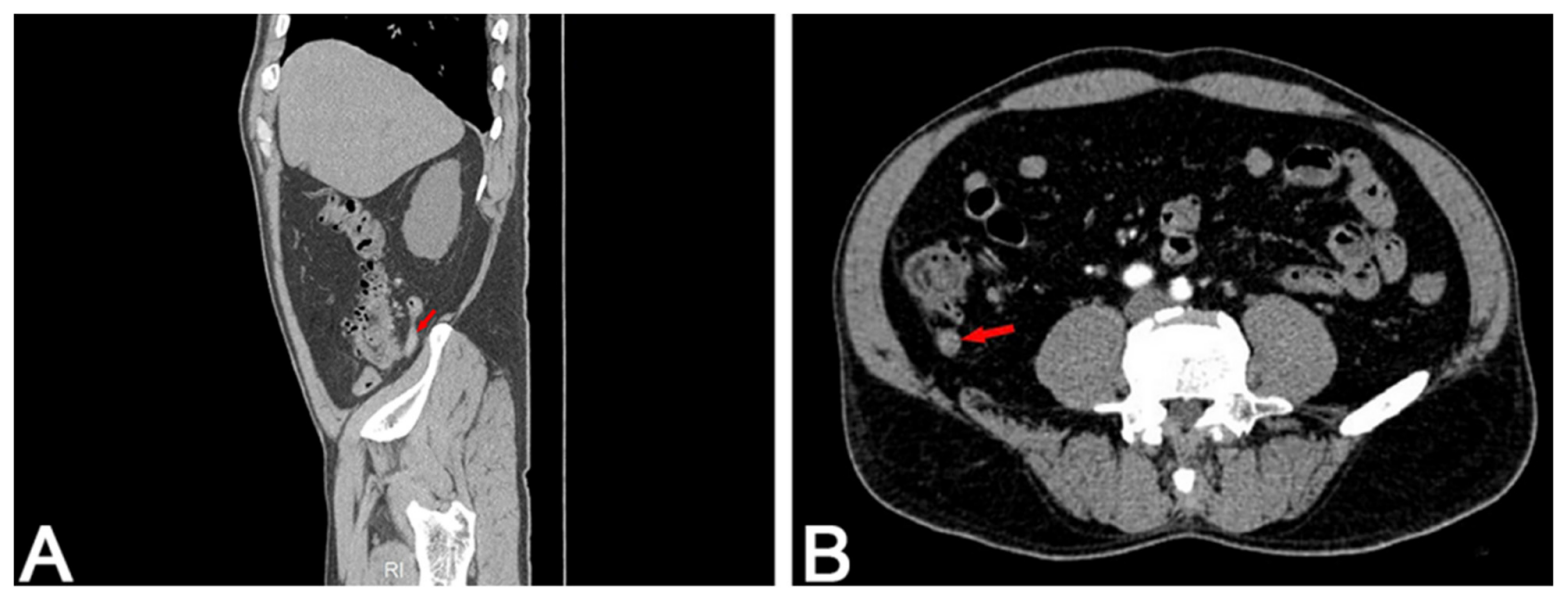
Contrast-enhanced abdominal CT scan. (A) Sagittal reconstruction shows an enlarged appendix with a maximal diameter of approximately 10 mm. (B) Axial view reveals a thickened appendix without evidence of acute appendicitis or surrounding fat stranding.
